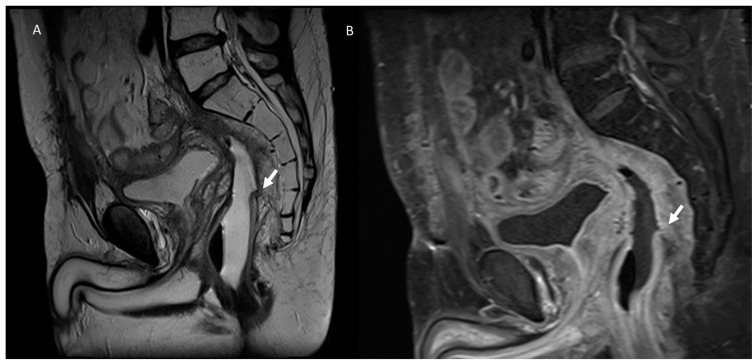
T2-W in sagittal plane (A) and T2-W in axial plane (B) MRI assessment of post-surgical fistula with sacral plane (arrows).
